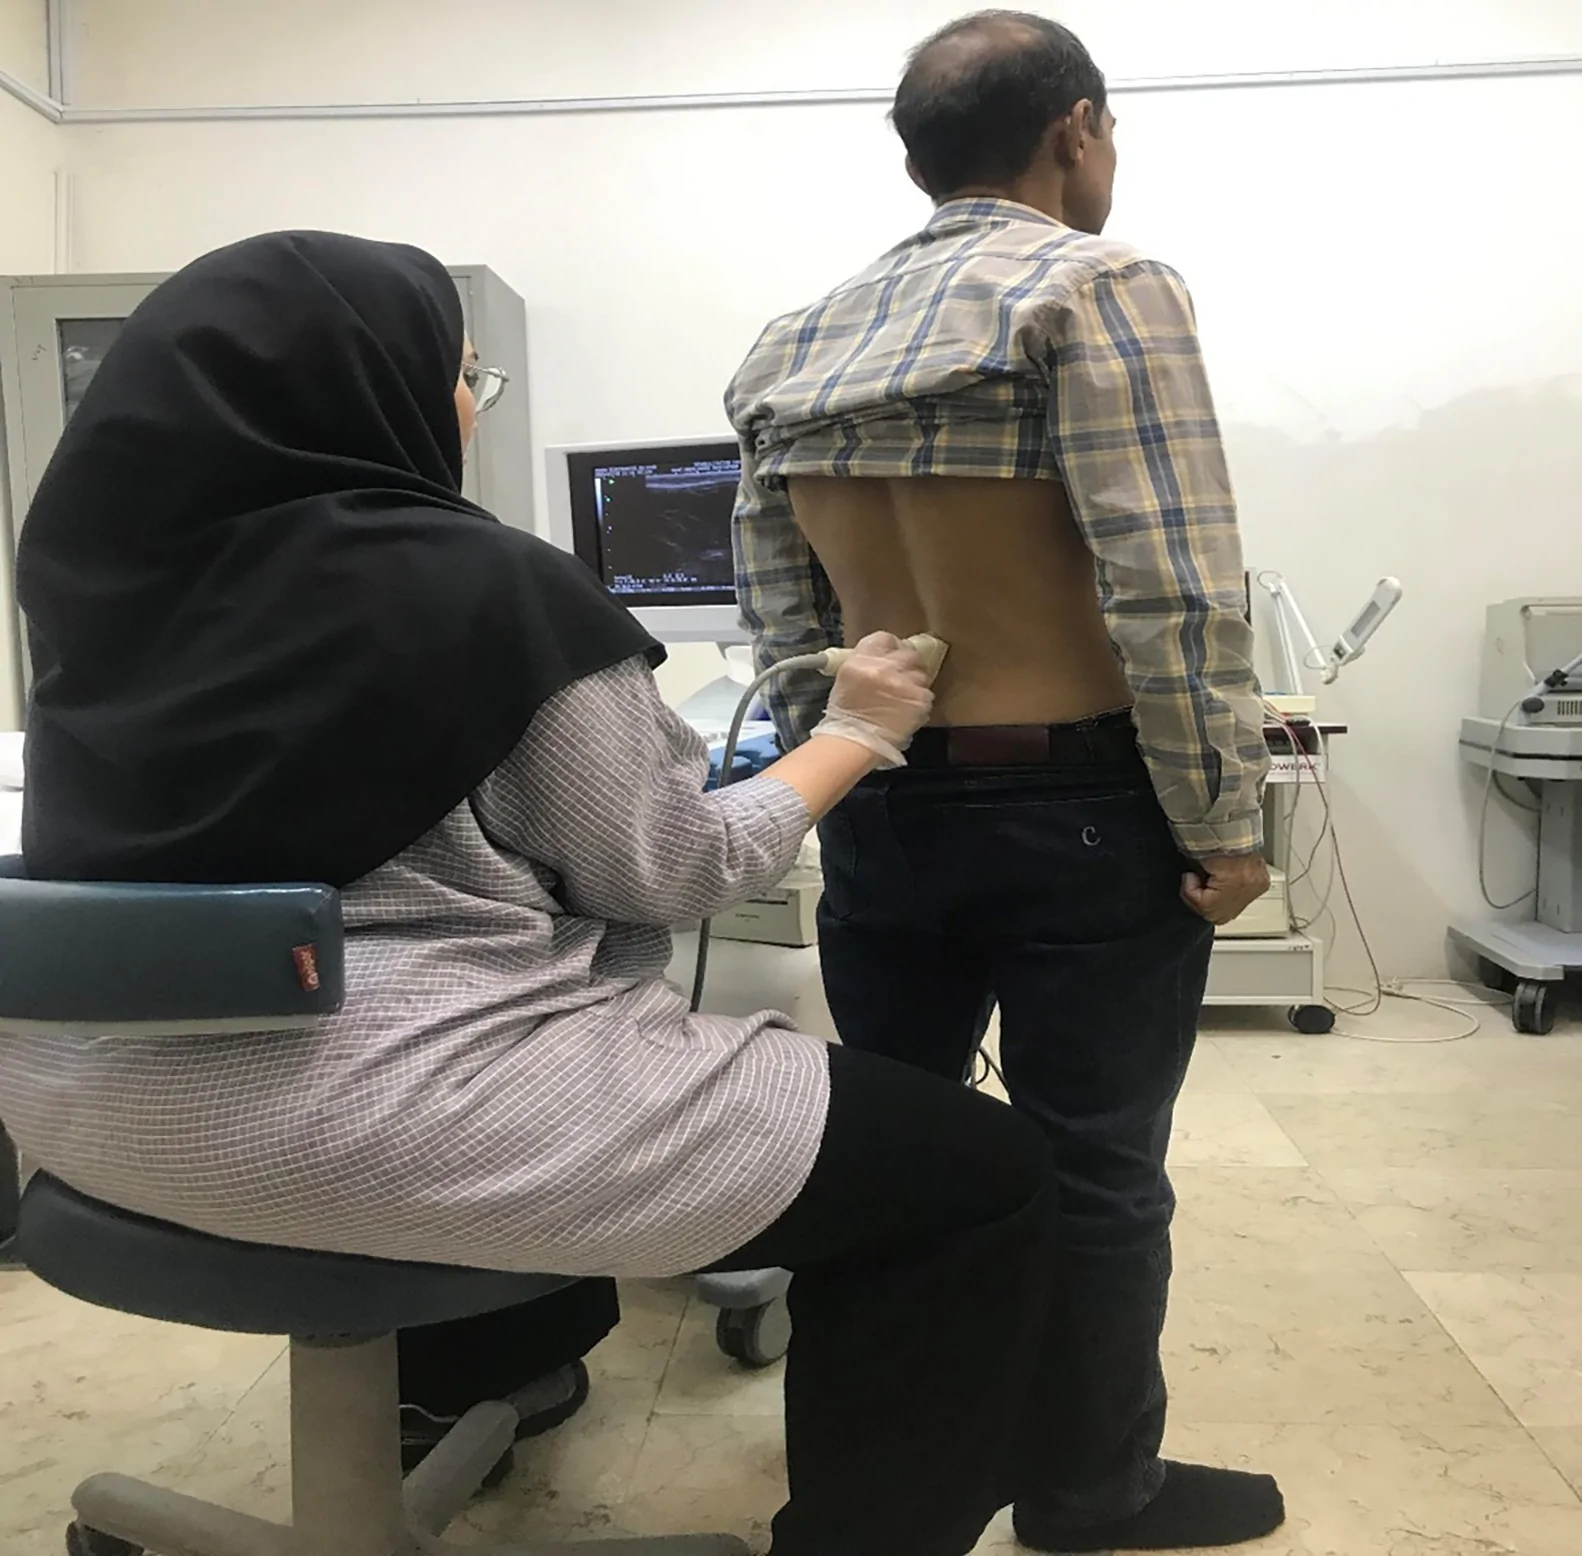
Ultrasound image showing the Multifidus muscle thickness in the standing position. Created by the authors. Published under CC BY 4.0.
